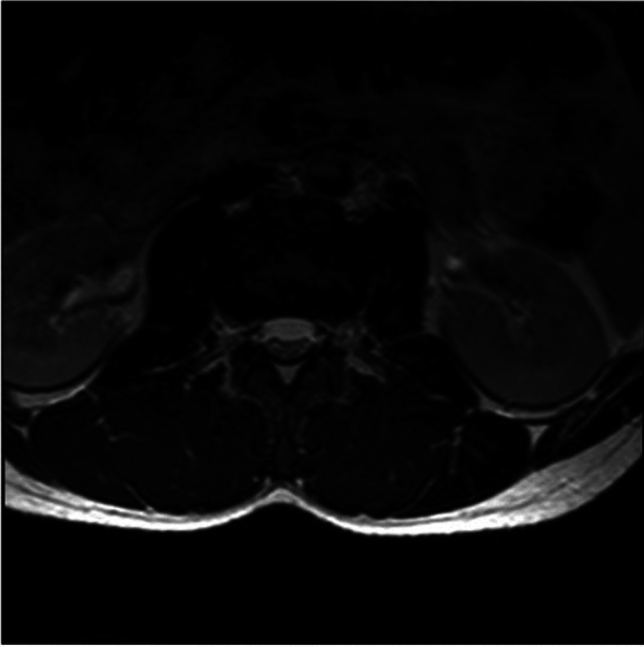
Axial MRI scan for lumbar disk herniation.
